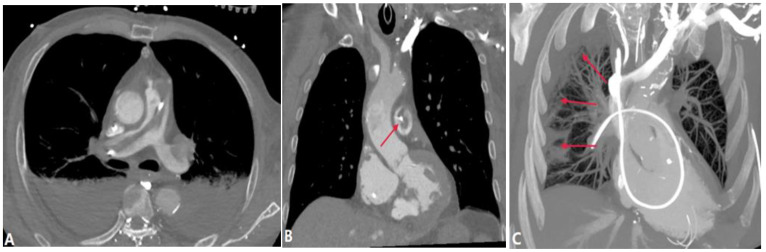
Catheter-related thrombosis in a patient post-CABG. (A). Axial CTA image shows filling defects extending from the main pulmonary to the right pulmonary artery along with the catheter. (B). Coronal CTA image shows the thrombus wrapped around the catheter (arrow). (C). Maximum-intensity projection image shows the path of the catheter and the peripheral consolidation, which is consistent with pulmonary infarction (arrows). CABG—coronary artery bypass grafting; CTA—computed tomography angiography.
